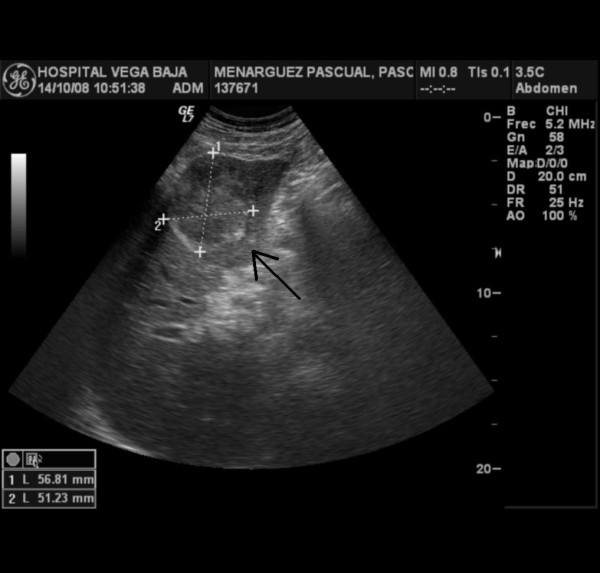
Abdominal ultrasonoraphy showing liver with muliple nodules, biggest in left liver lobe (56 × 51 mm.), highly suspicious for hepatocellular carcinoma.
